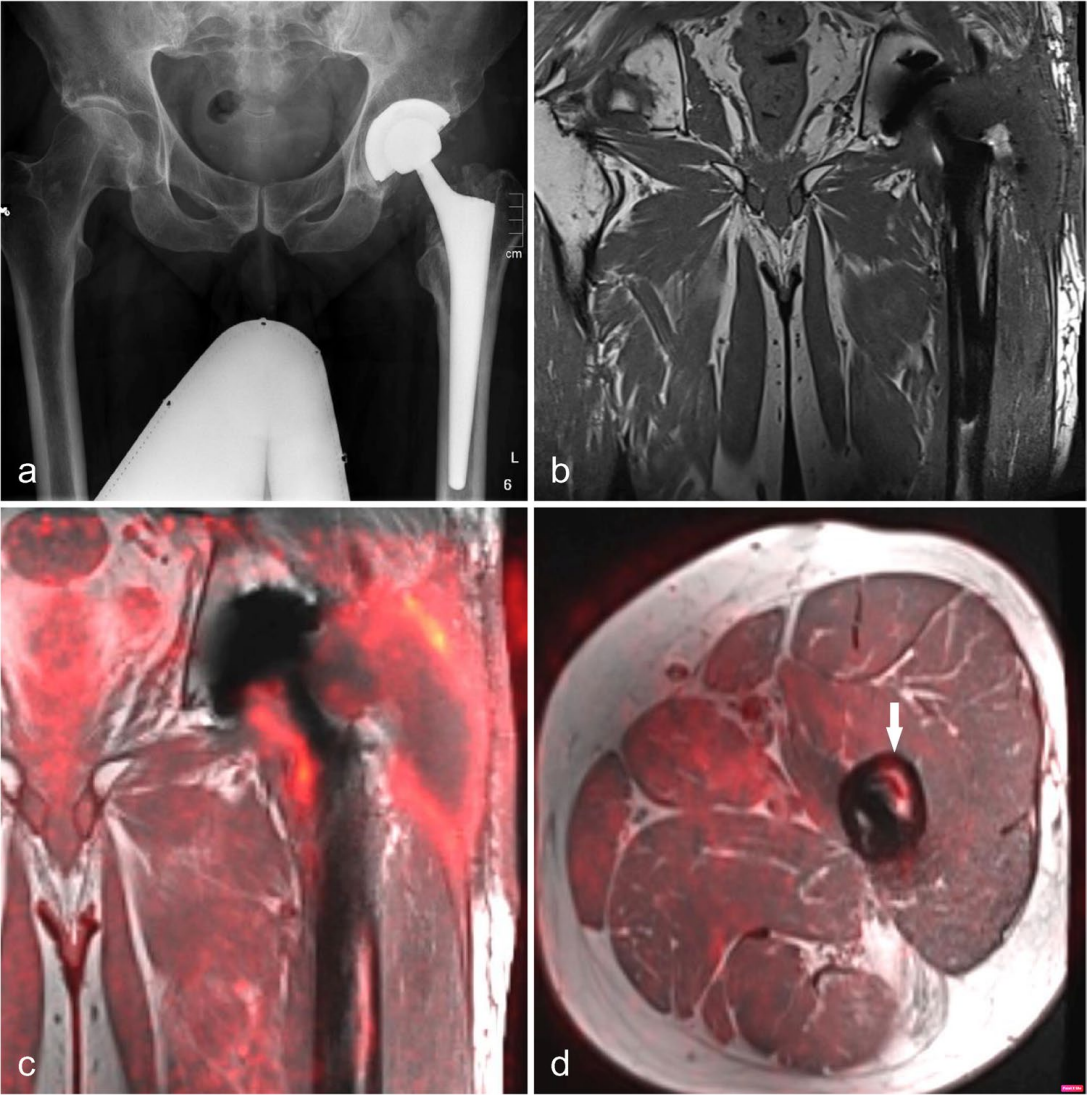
A X-ray of a 54-year-old man with left-sided hip prosthesis. B Coronal T1-weighted magnetic resonance imaging (MRI) shows distinct subfacial fluid collections extending around the prostheses neck. Fused.^18^F-FDG-PET/MRI (C + D) shows an elevated tracer uptake around the prosthesis neck, shaft, and soft tissue. The increased uptake in the periprosthetic bone marrow of the femur (arrow) was confirmed as inflammatory involvement by prosthesis revision and sonication
